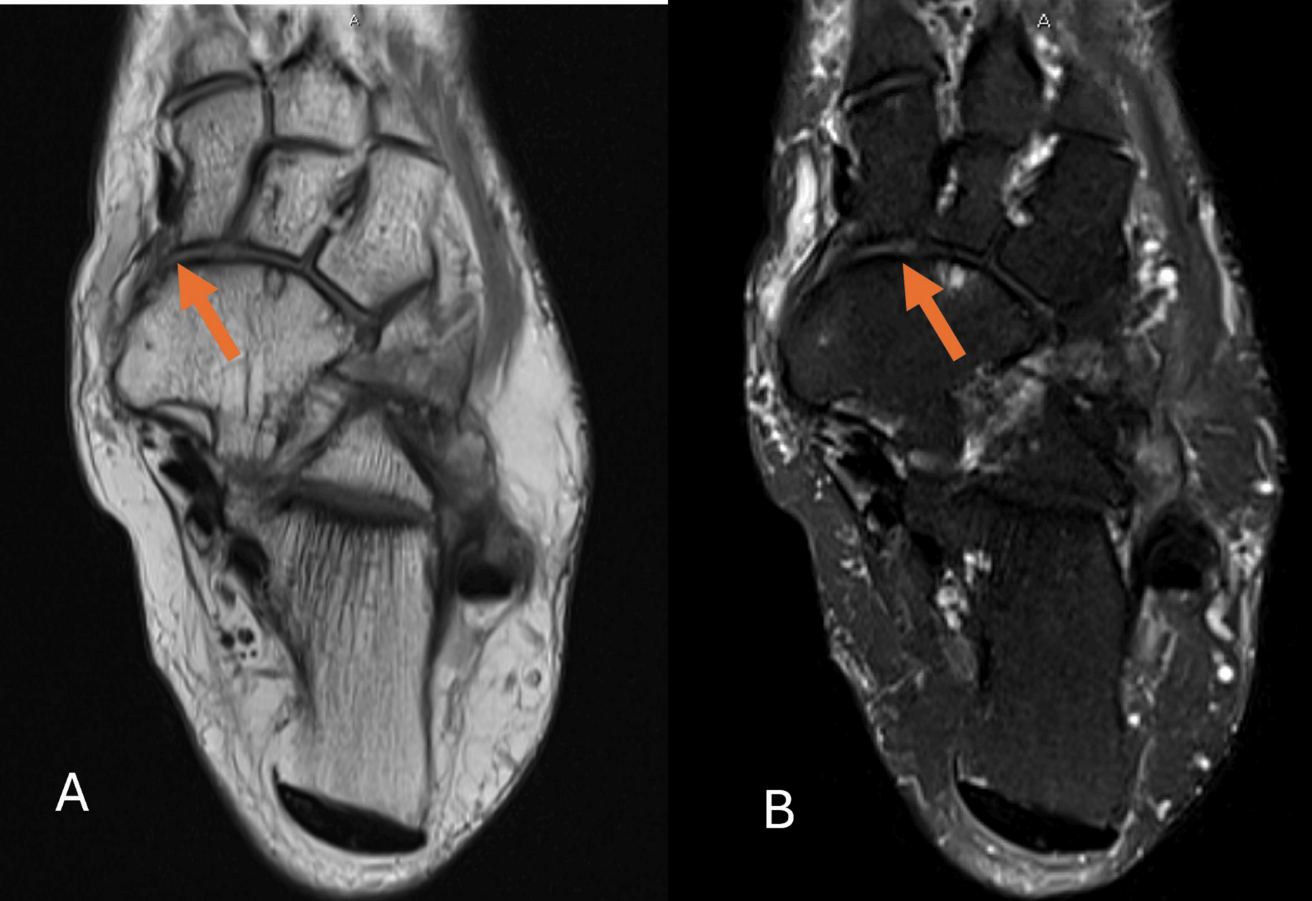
(A) Proton density axial-weighted MRI and (B) T2 fat-saturated axial-weighted MRI showing the congenital absence of the navicular bone with talo-cuneiform articulation and osteoarthritic changes (arrows) MRI: magnetic resonance imaging
